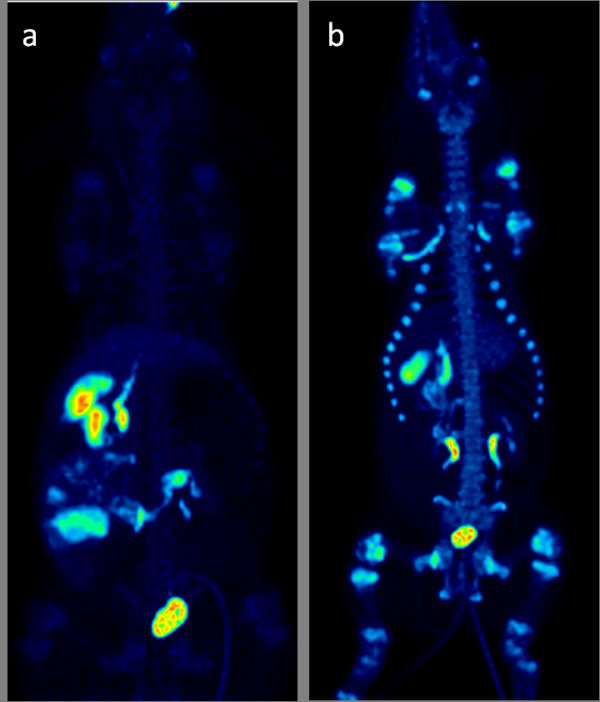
Three-dimensional maximum intensity projection. The figure shows 3-D maximum intensity projection 90 min after administration of (a) [^18^F]FE-DTBZ-d4; low accumulation in bone structures indicates low levels of free [^18^F]F^- ^and (b) non-deuterated [^18^F]FE-DTBZ; high accumulation in bone structures is due to higher levels of free [^18^F]F^-^. Colors indicate SUV 0 (black) to SUV 30 (white).
